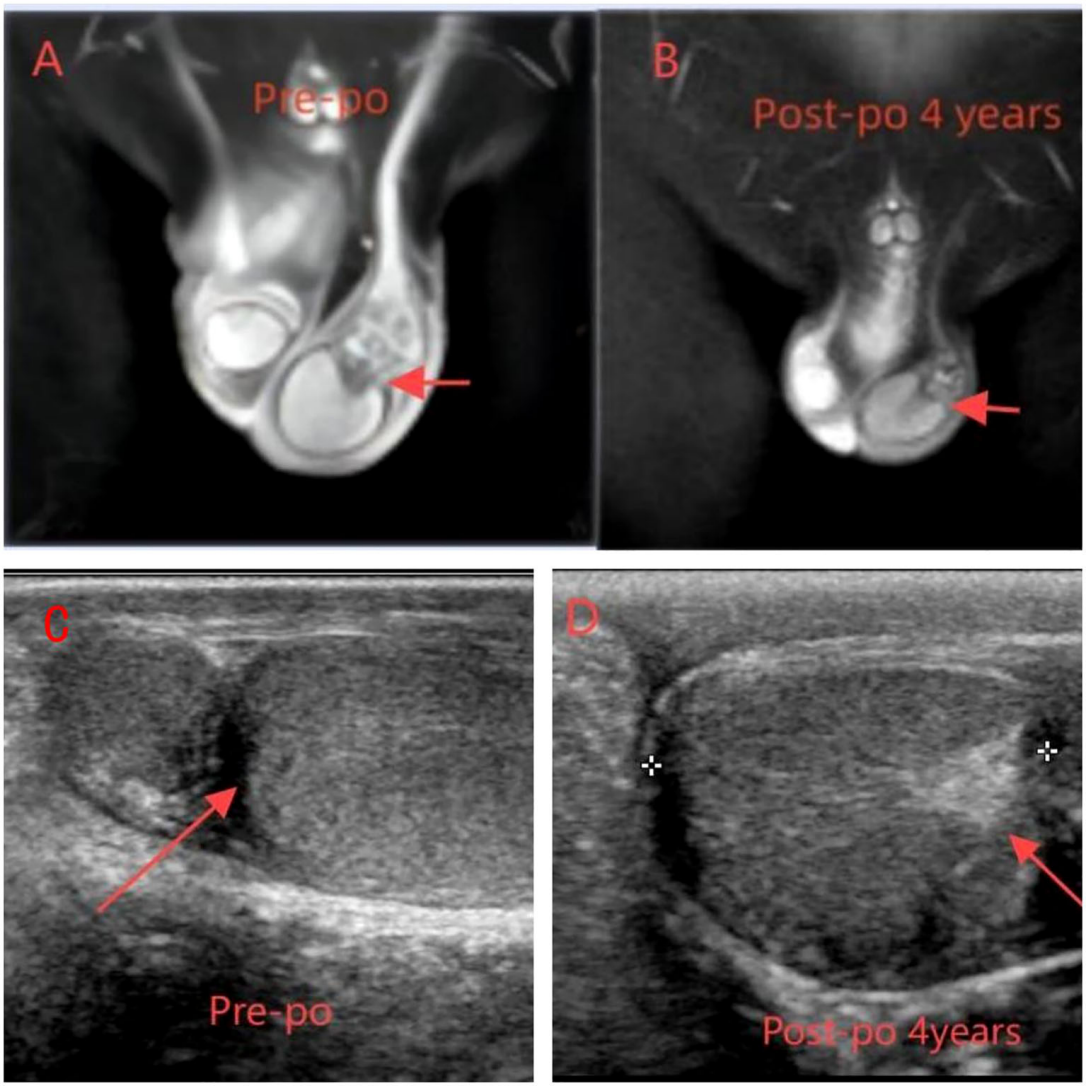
(A, C) MRI and ultrasound preoperation (A) On the outer side of the left testicle, a mixed signal mass of 13 × 14 mm was visible, with T2W1 sequence signals lower than the adjacent testicle. Mild enhancement was observed after contrast administration. (C) Enlargement of the left testis with slightly increased parenchymal echogenicity and globular thickening of the epididymal head. (B, D) MRI ultrasound postoperation (B) The left epididymal mass was less obvious than it was before the surgery. There was still a wedge-shaped low signal on the outer side of the testicle. (D) The left testis is enlarged and exhibits enhanced parenchymal echogenicity.
